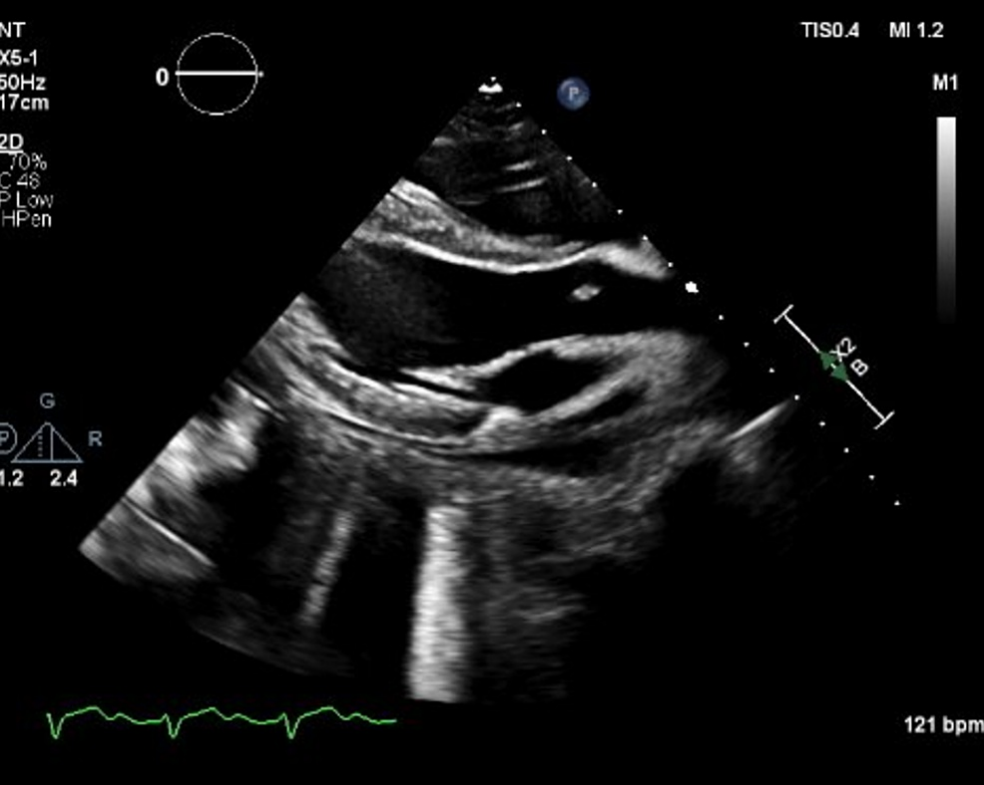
Transthoracic echocardiogram.
